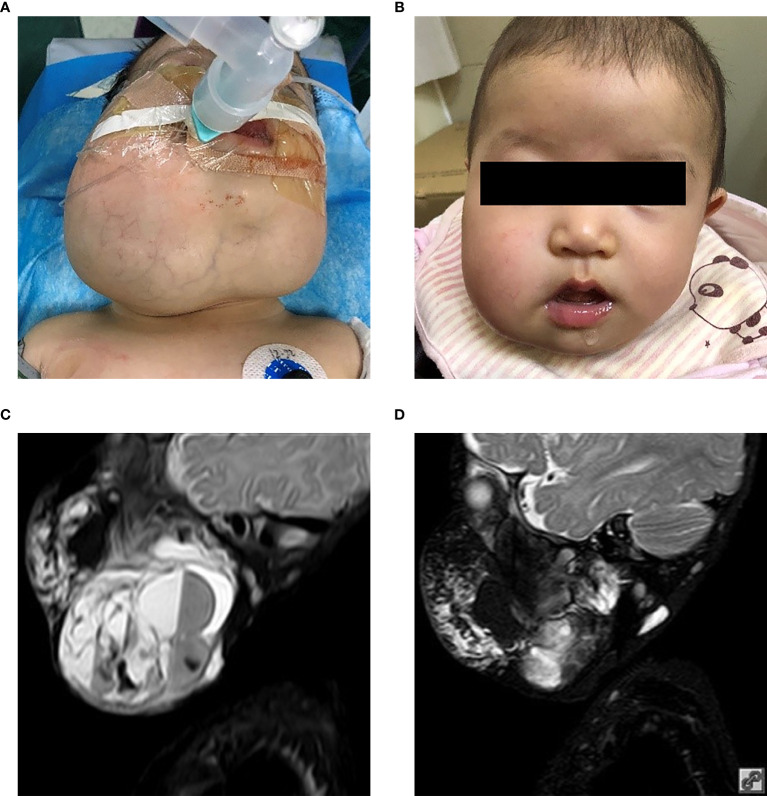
Patient 2 from Table 1. Extensive mixed, mostly macrocystic cervicofacial lymphatic malformations requiring intubation at birth. (A) Baseline after sclerotherapy, prior to initiation of sirolimus. (B) Five months on sirolimus. Sirolimus initiated at 5 months of age. T2 magnetic resonance imaging upon (C) initiation of sirolimus and (D) response after 5 months. T2, transverse relaxation.
